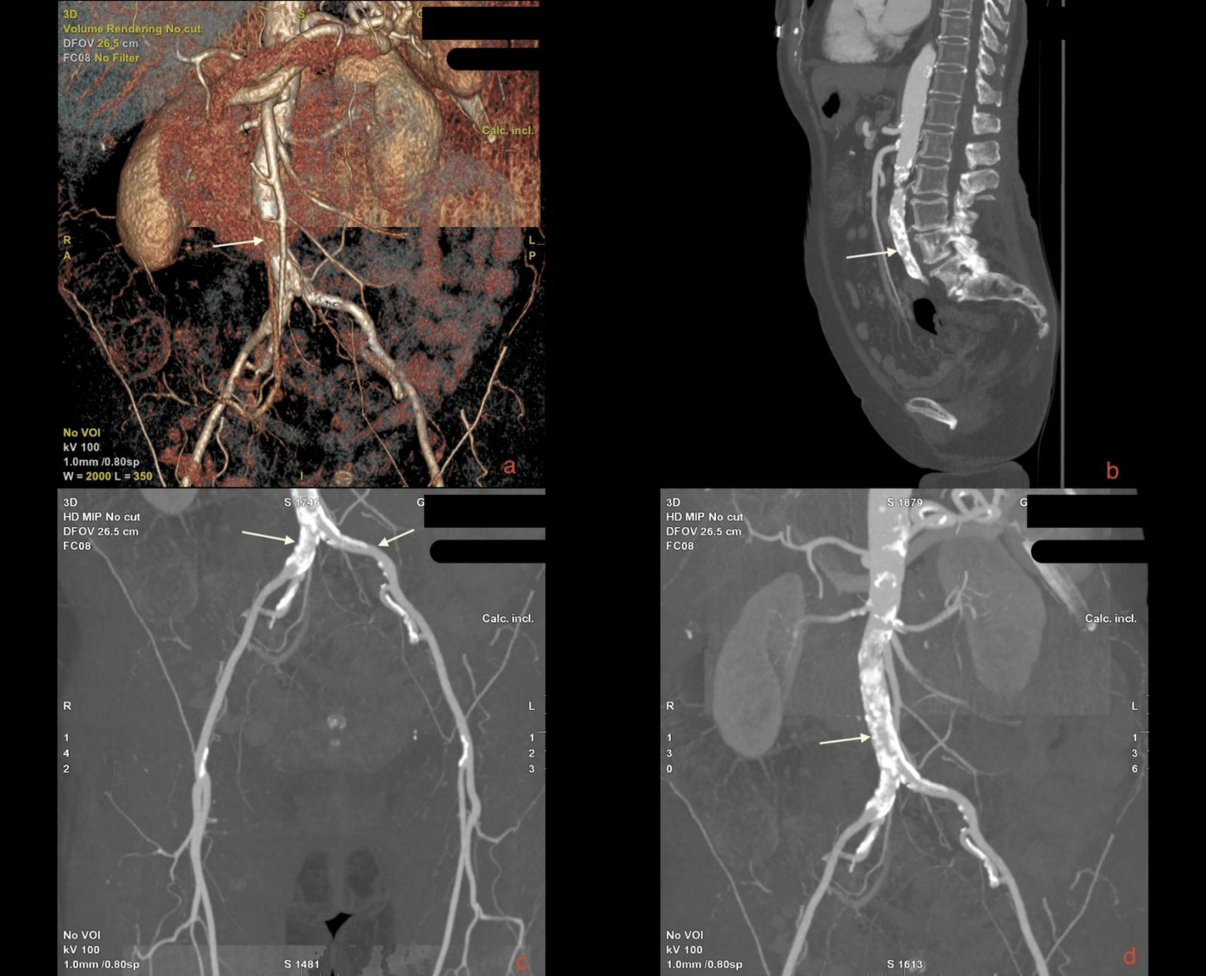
Multiplanar and 3D CT angiographic reconstruction of infrarenal aortoiliac occlusive disease (Leriche syndrome). (a) 3D volume-rendered reconstruction shows a tight infrarenal aortic stenosis with extensive calcifications and collateral vessel development. (b) Sagittal reformatted CT image demonstrates a heavily calcified mixed plaque causing a severe infrarenal aortic stenosis. (c) Coronal maximum intensity projection (MIP) of the iliac and femoral arteries shows reduced perfusion bilaterally with patent distal runoff. (d) MIP image of the abdominal aorta confirms a long-segment calcified stenosis of the infrarenal aorta, consistent with a TASC II type D lesion. Arrows indicate key calcified segments and zones of critical luminal narrowing. TASC II: TransAtlantic Inter-Society Consensus II.
